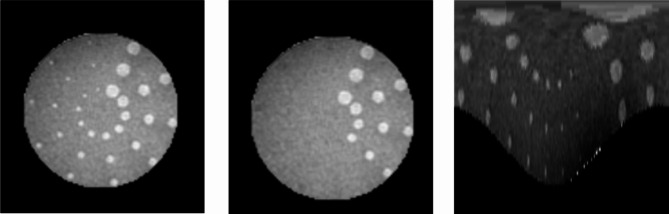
A) original MR image for the low contrast. B) image with 6 spokes masked. C) image with pixels transformed from cartesian to polar coordinates
